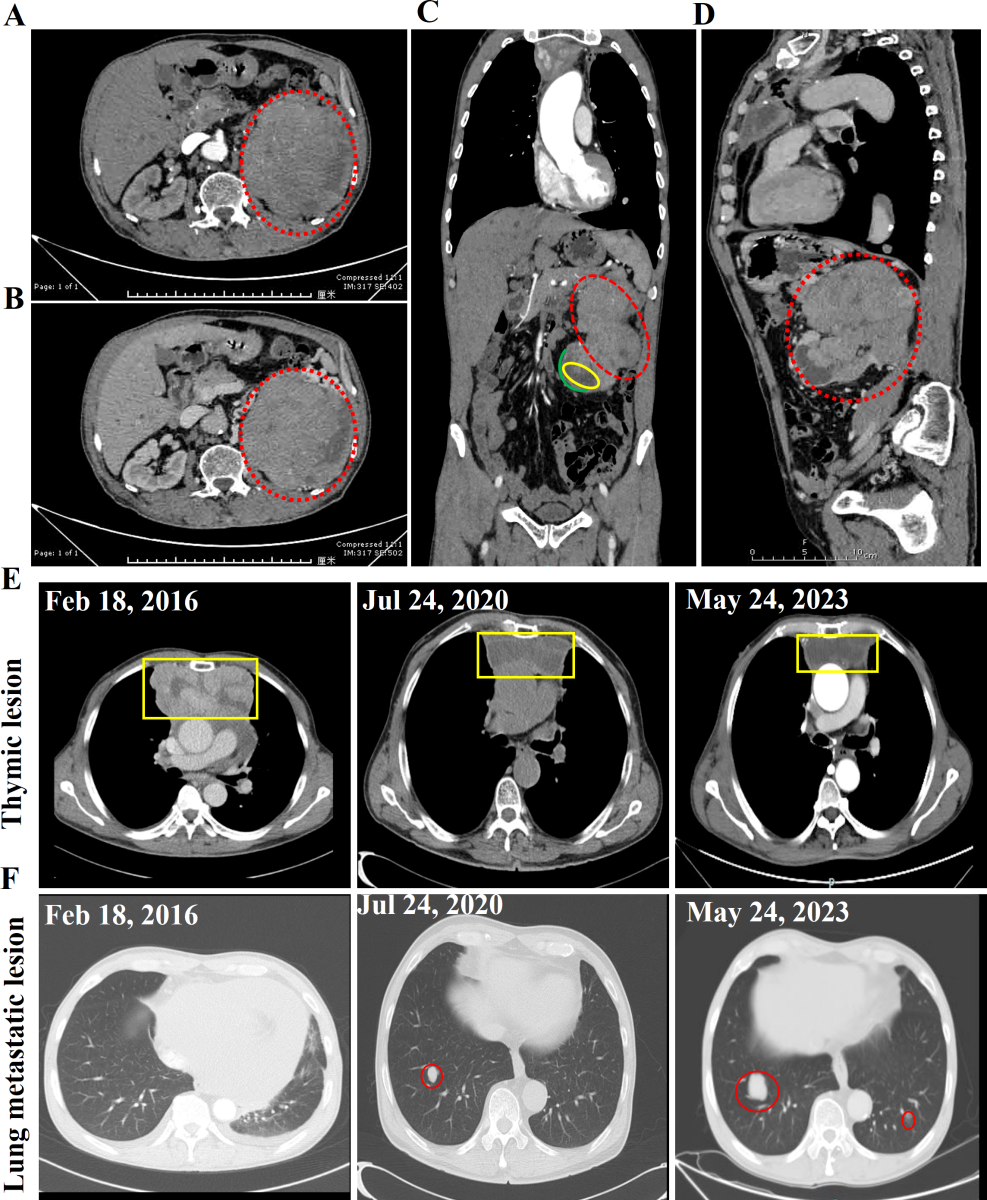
Abdominal contrast-enhanced CT scan images of this case. The normal morphology of the left kidney is absent, and a large, heterogeneously enhancing mass is observed, with the degree of enhancement being most prominent in the venous phase. The largest cross-sectional area of the lesion measures approximately 12 cm × 11 cm, and multiple tortuous vascular shadows are seen surrounding the lesion. (A) Arterial phase. (B) Venous phase. (C) Coronal planes. (D) Sagittal plane. The dotted red line indicates the thymoma renal metastasis tumor. The yellow box highlights the hydronephrosis, while the green line delineates the renal cortex. (E) Enhanced CT images of the thymic lesion (E) and lung metastatic lesion (F) at different time points. The yellow box indicates the thymoma lesion, while the red circle denotes the lung metastatic lesion.
